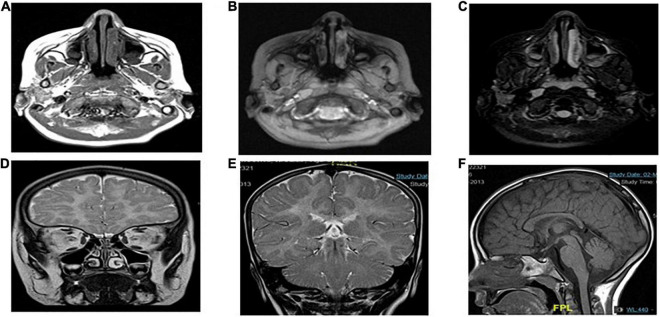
MRI showing the characteristics of *POLR1C* related leukodystrophy caused by POLR1C variant. Axial T2-weighted (A,B,D,E) and sagittal T1-weighted (F) images of case at the age of 4 years at the time of MRI.
